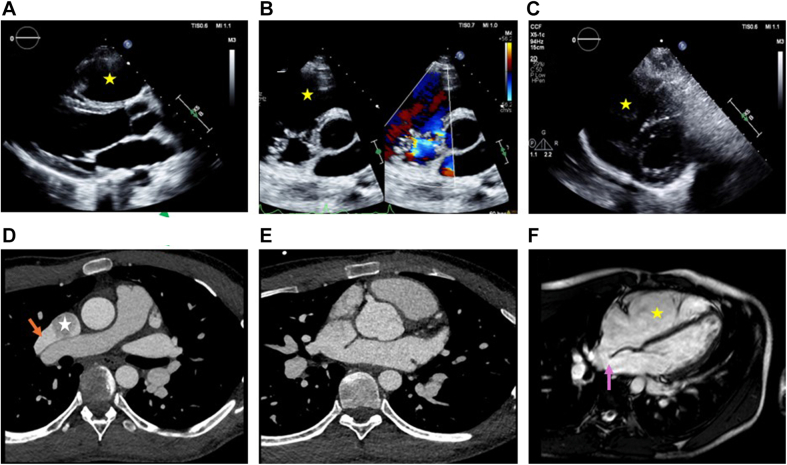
Preoperative Evaluation of Sinus Venosus Atrial Septal Defect and Partial Anomalous Pulmonary Venous Return (A) Parasternal long-axis view on transthoracic echocardiography showing right ventricular dilation (yellow star); (B) short-axis view on transthoracic echocardiography showing mild tricuspid regurgitation (red arrow) and dilated right ventricle (yellow star); (C) short-axis view on transthoracic echocardiography showing significant right ventricular dilation (yellow star); (D) right upper pulmonary vein (orange arrow) draining into the superior vena cava (white star); (E) right middle pulmonary vein draining into the right atrium at the level of the superior sinus venosus defect (F) cardiac magnetic resonance imaging showing the superior sinus venosus defect (purple arrow) and a dilated right ventricle (yellow star). CCF = coronary cameral fistula.
